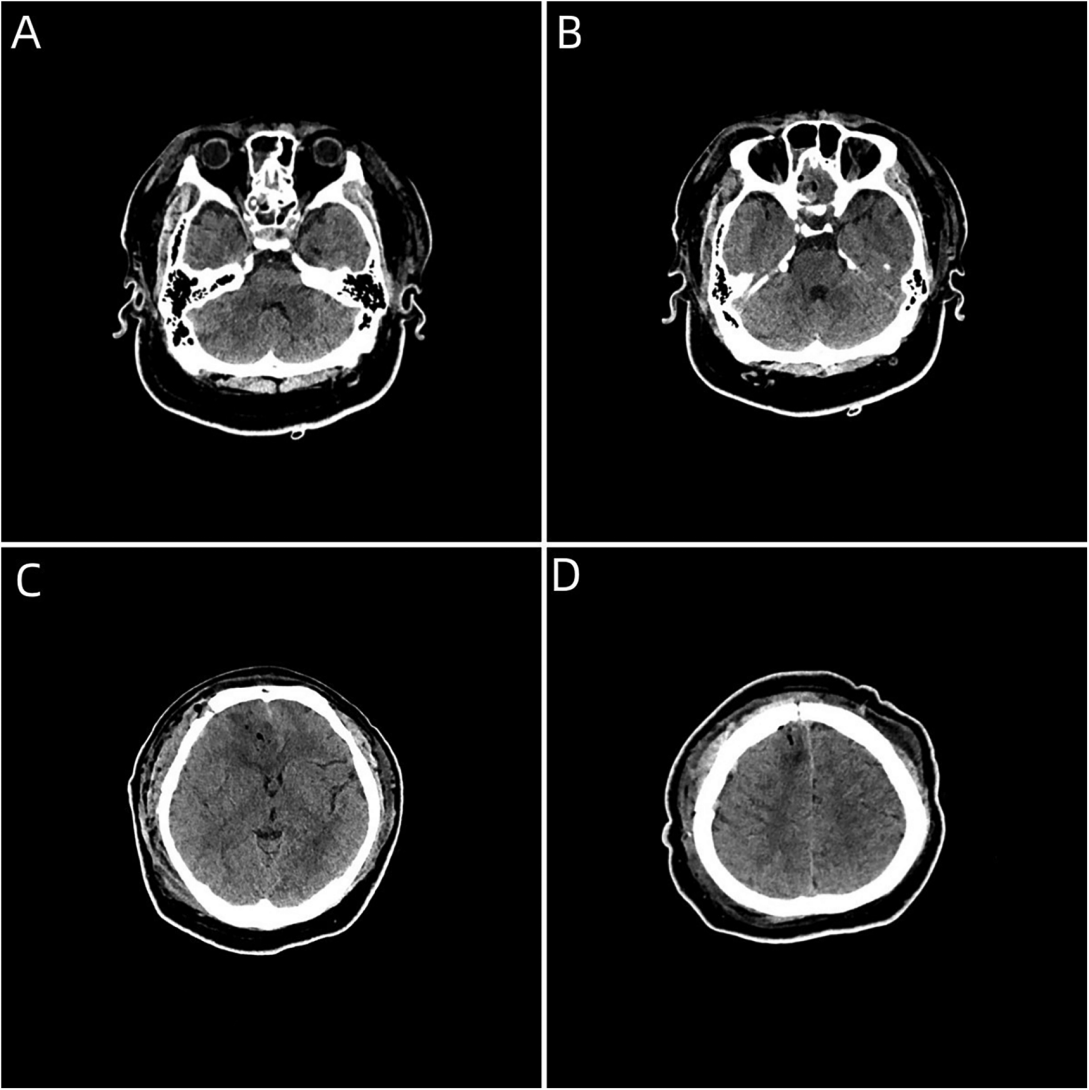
(A–D) Postoperative craniocerebral CT image showing complete removal of the intracranial foreign body, without foreign body residue or secondary bleeding complications.
